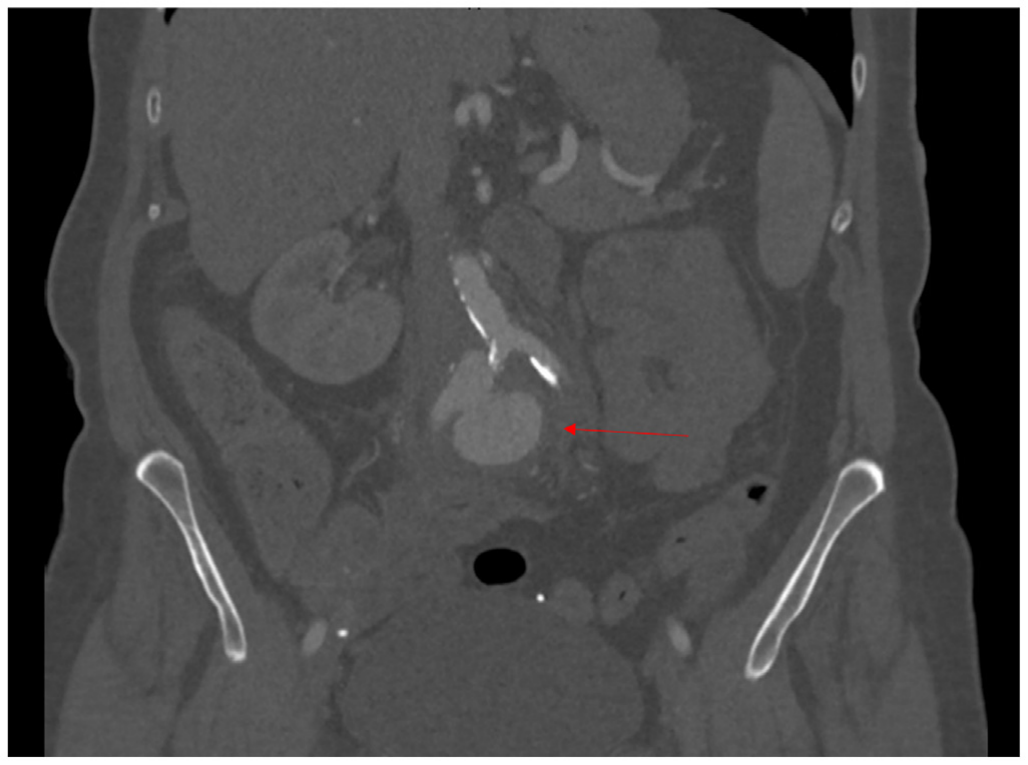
Computed tomography scan of right common iliac pseudoaneurysm (PSA) (red arrow).
